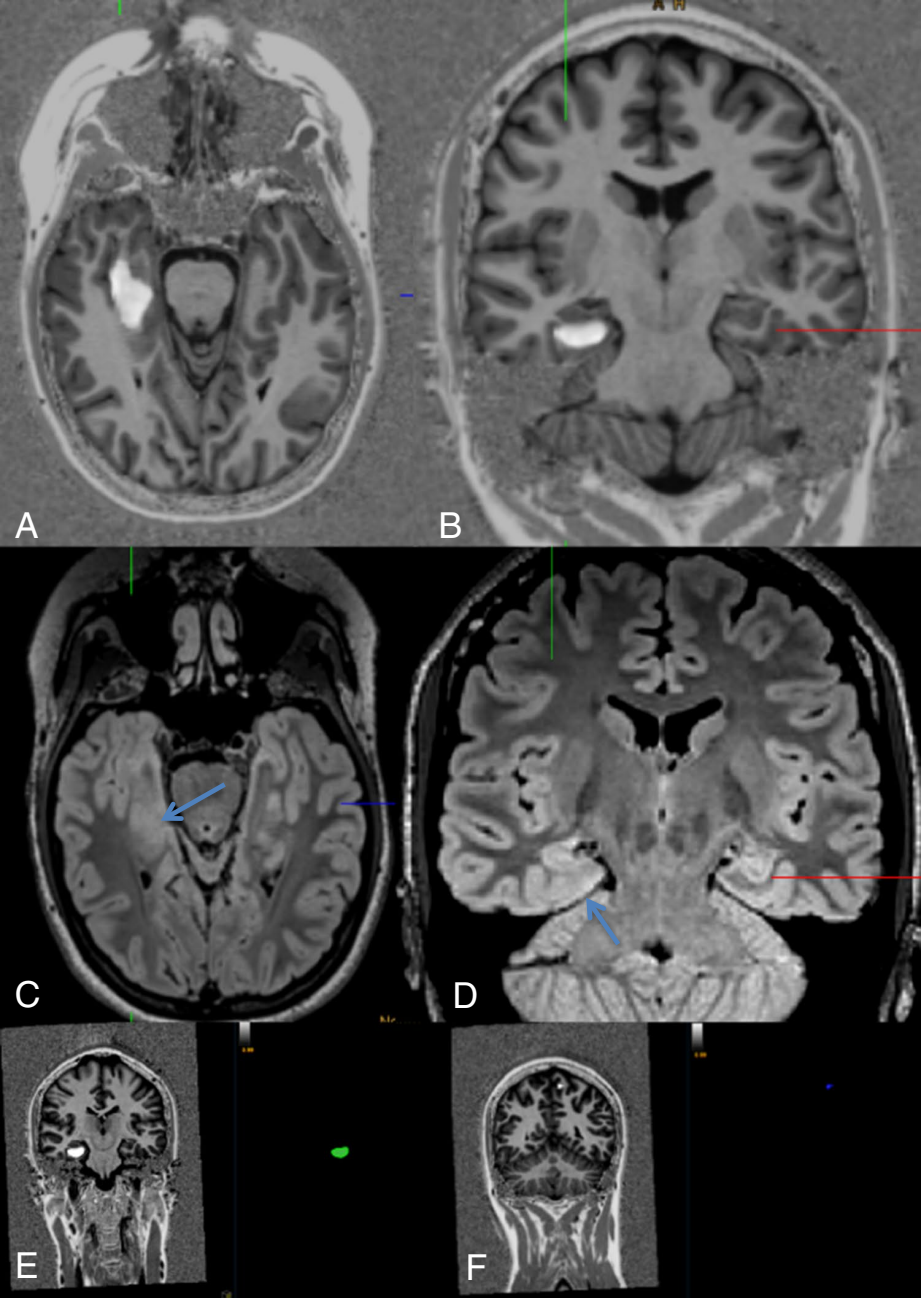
A 22-year-old woman with auditory, somatosensory, and visual auras evolving to bilateral tonic–clonic seizures. Postictal hemianopia to the left side (#22). Scrolling through the co-registered MP2RAGE data set led to the identification of an FCD of the right parahippocampal gyrus (A, B). Axial (A) and coronal FLAIR images showed a corresponding subtle blurring of the subcortical white matter (C, D). Intracranial stereo-EEG proved the lesion to be epileptogenic. Lesion volume was 1.6 ml (E) with a tiny false-positive lesion of the left superior frontal gyrus (0.02 ml) (F)
